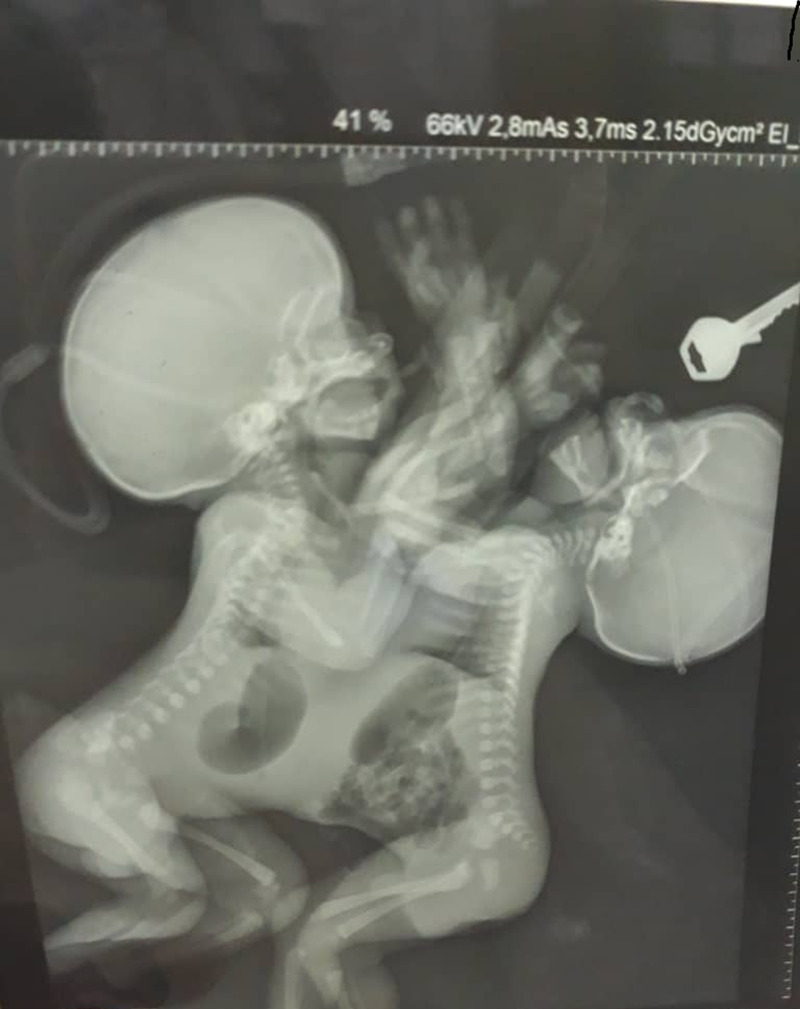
radiographie ASP (abdomen sans préparation) des jumeaux conjoints montrant des anses intestinales déviées à gauche
